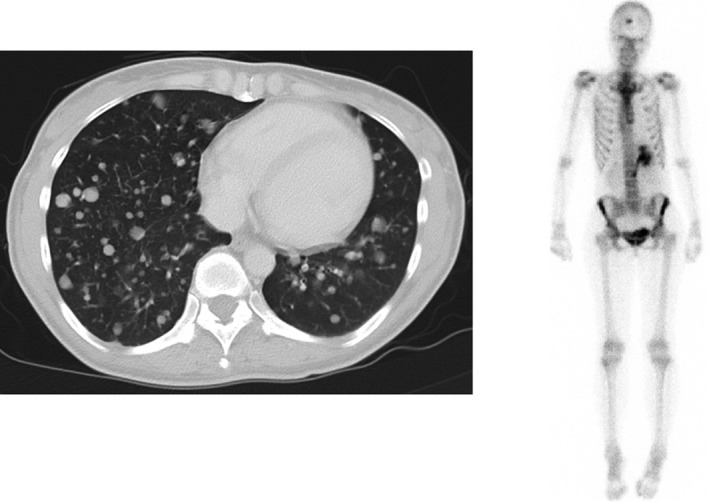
Computed tomography and bone scintigraphy showed multiple lung and bone metastases.
